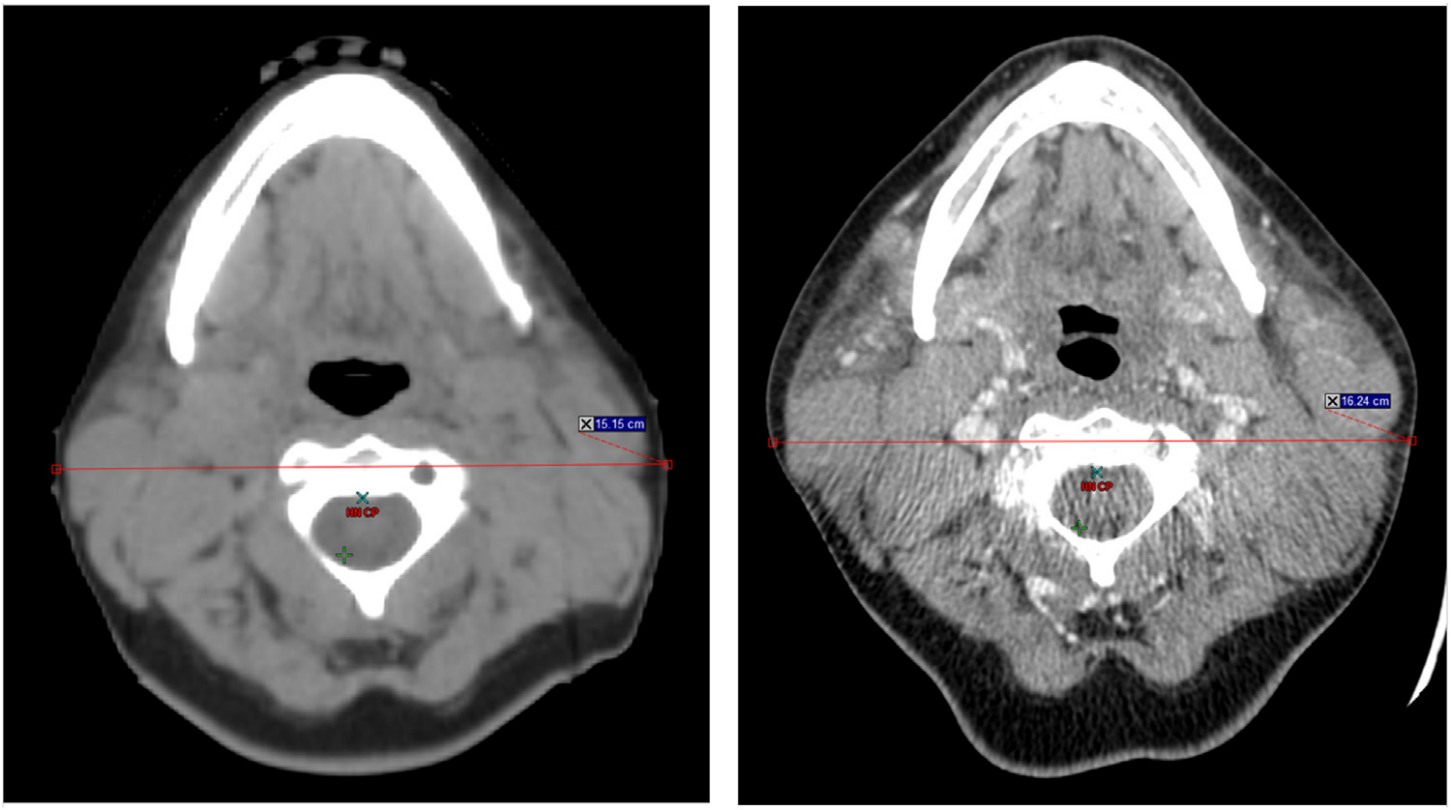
Computed tomography scan of neck before radiation therapy (left) and after radiation therapy (right). Before, neck diameter was 15.15 cm. After, it was 16.24 cm with marked change in the shape of soft tissues.
